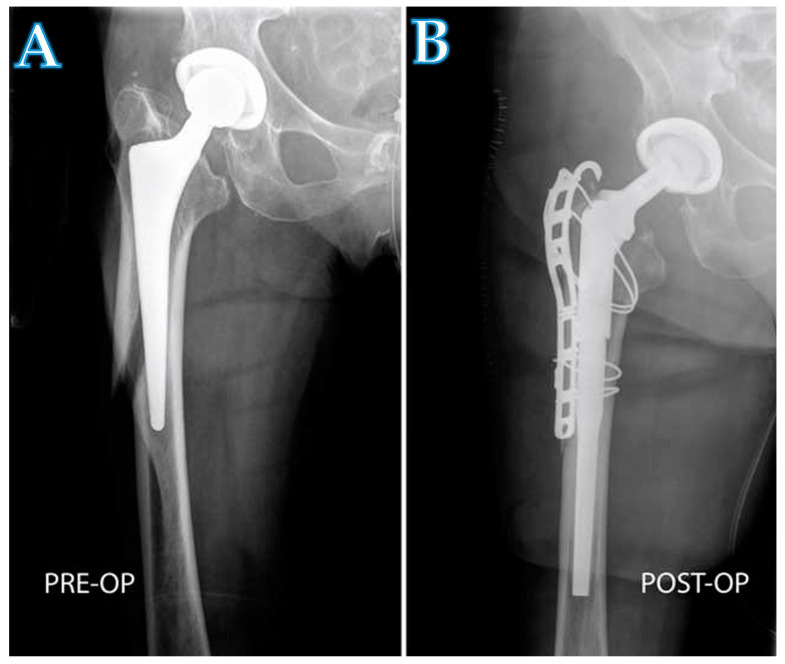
A radiograph showing a patient with a periprosthetic fracture (A), and the same patient after being treated with a modular stem (B). The implantation of the long modular stem and the reduction of the fracture was performed with a proximal and distal extension of the DAA.
